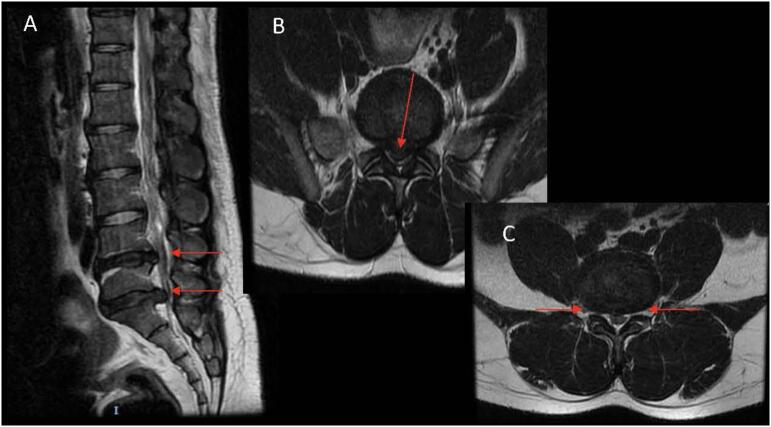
Patient 4: Preoperative MRI. (A) Sagittal T2-weighted MRI showing disc protrusion and L4–5 and disc extrusion at L5-S1. (B) Axial T2-weighted image showing stenosis at L4–5. (C) Axial T2-weighted image showing stenosis at L5-S1.
